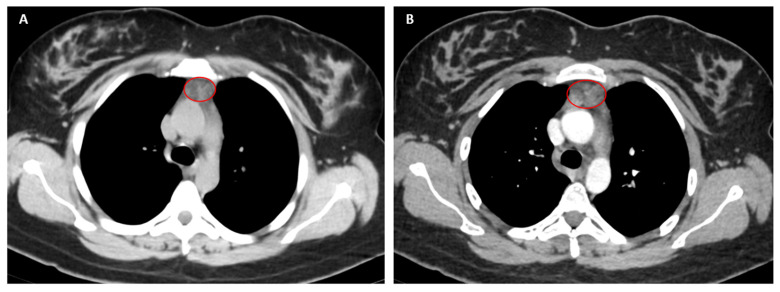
CT scan was performed two months after adrenalectomy and showed no right adrenal remnants/recurrence, neither any lymph node involvement. Accidentally, a nodule of 3.3 by 1.8 cm was identified within the fat tissue component in the thymus area indicating a thymic remnant. This finding was not suspected for a thymus tumor/malignancy; thus, no surgical approach was recommended, only serial CT scan surveillance. [(A,B). CT scan: thymus remnant (red cercle). (A). native phase, axial plane; (B). arterial phase, axial plane].
